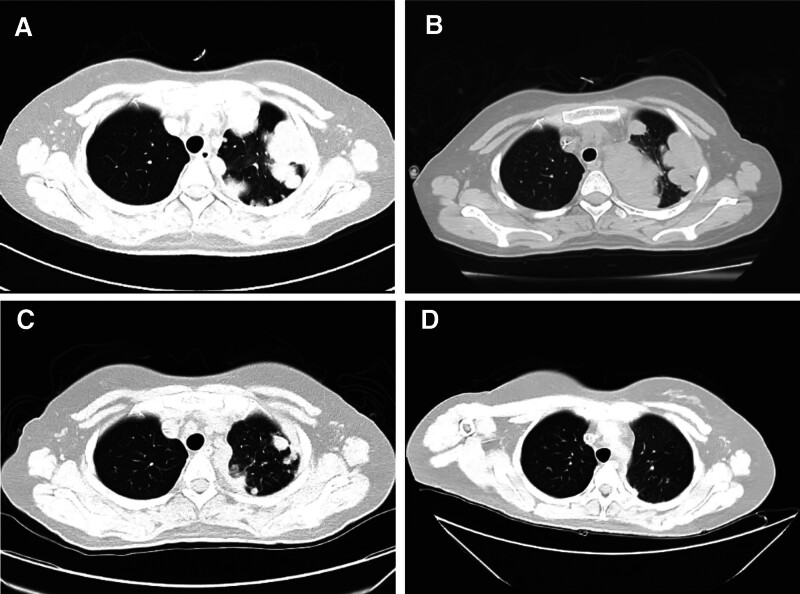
Computerized tomography (CT) scan results. (A) Initial chest CT revealed multiple high-density nodular shadows within the left lung and pleura. (B) A follow-up examination after 7 days demonstrated an increased occupation within the left lung, with the largest mass measuring of 5.2 × 3.6 cm.(C) A subsequent chest CT demonstrated a significant reduction in the high-density shadow range within the left upper lobe compared to the initial examination. (D) A follow-up chest CT revealed multiple varied-sized nodular with high-density shadows in the left upper lobe, some of which exhibited undefined boundaries with the pleura. The size and number of nodules significantly decreased and some of them exhibited cavitations.
